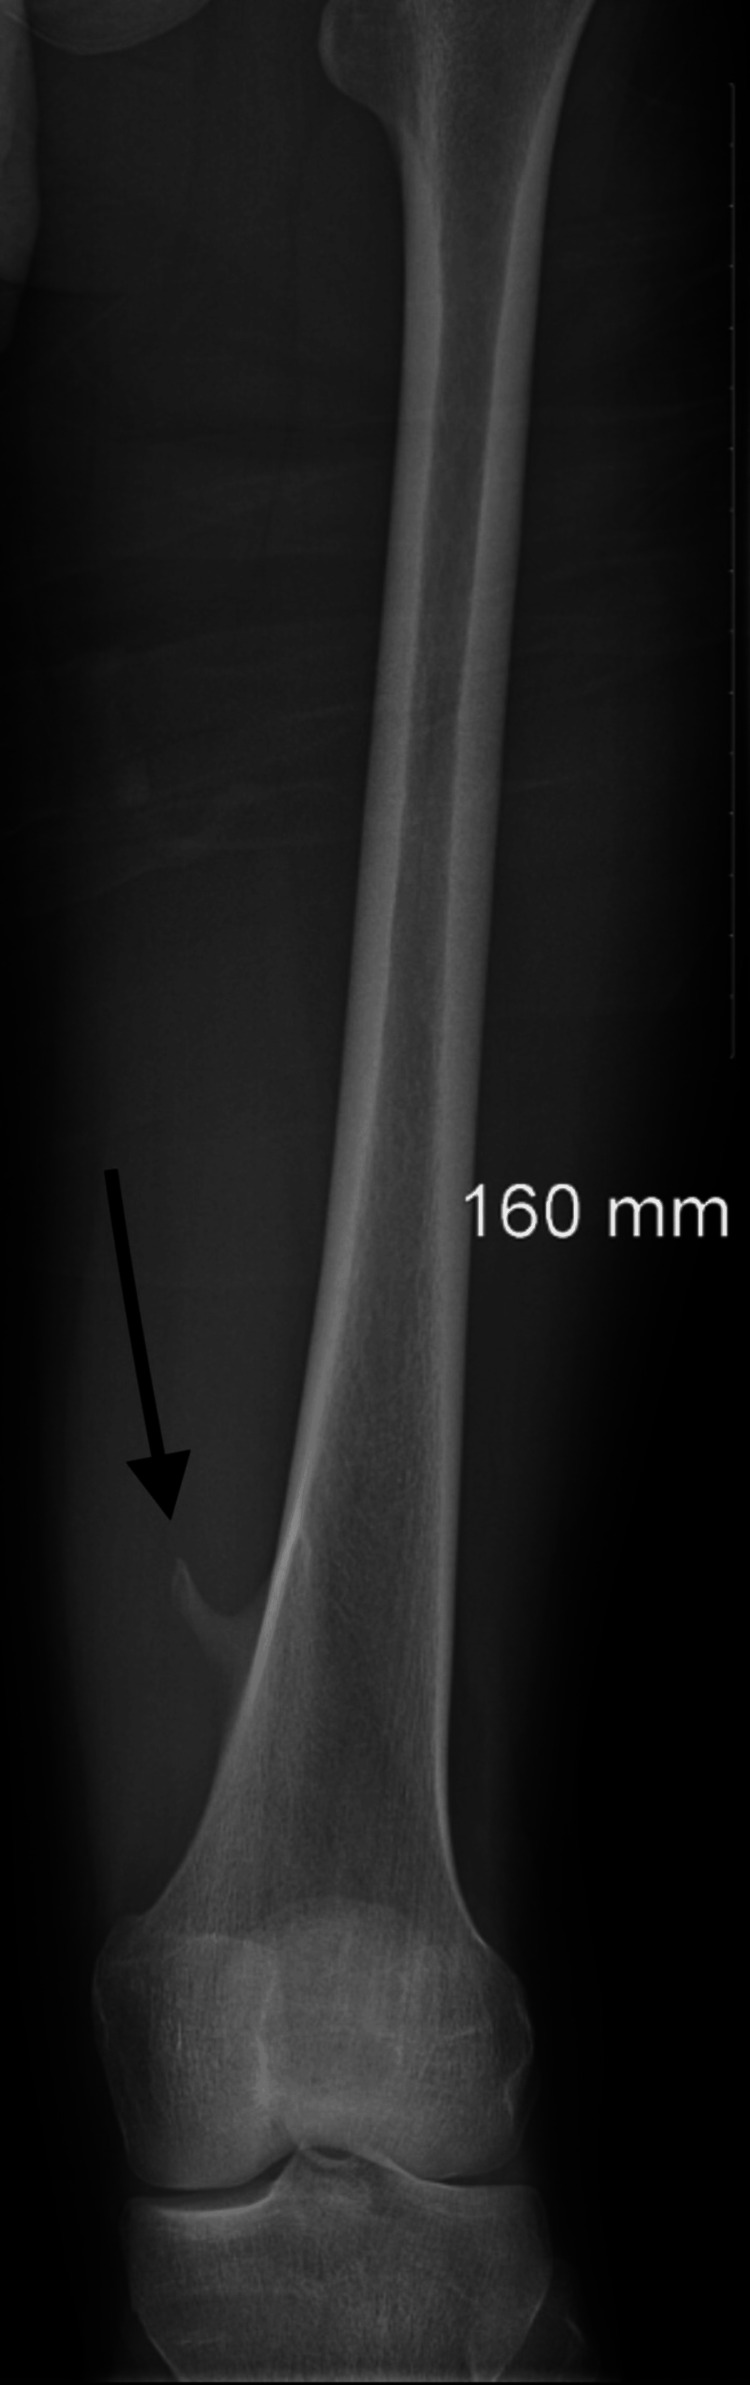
X-ray showing osteochondromas on the left distal femur posteromedially
